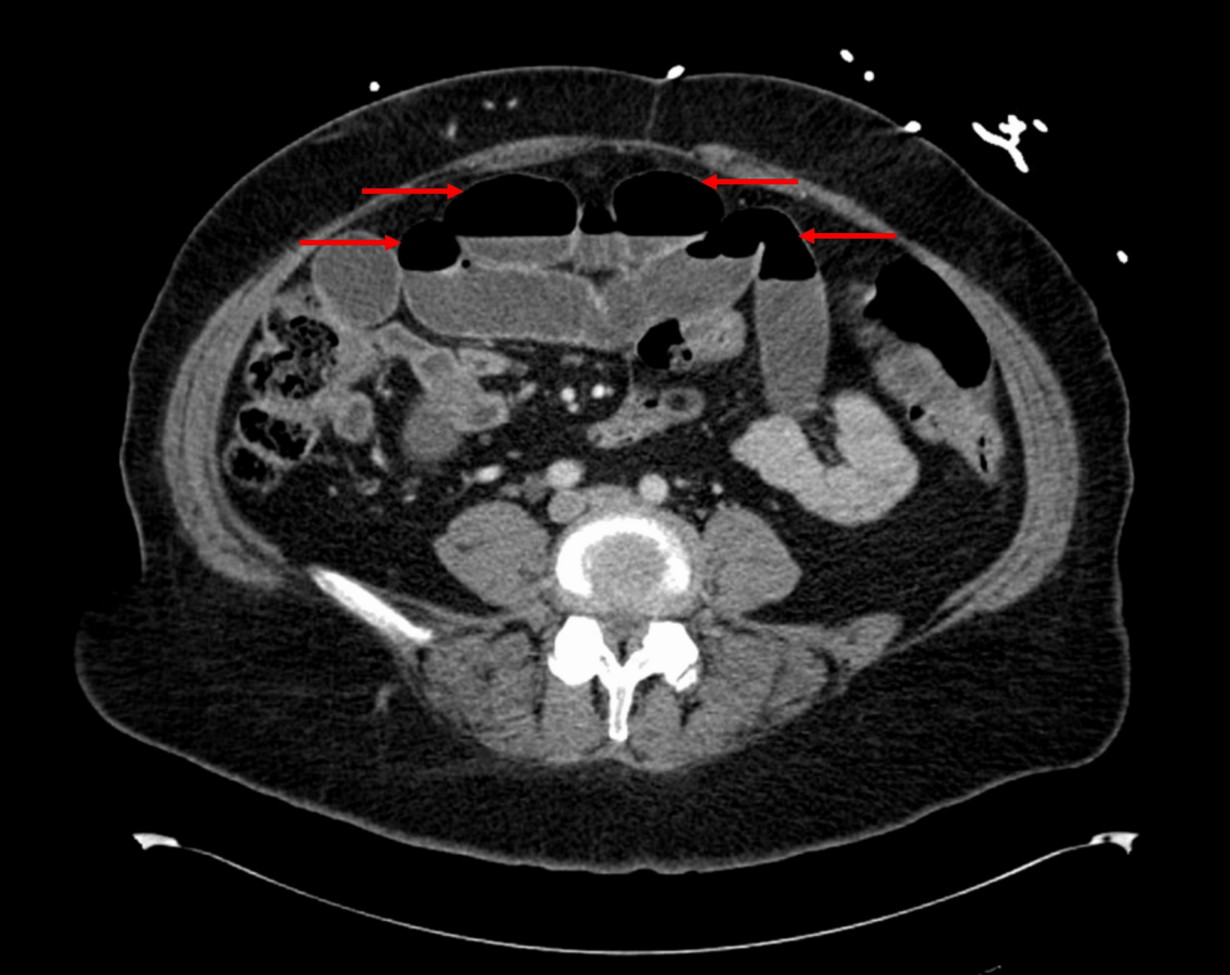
CT scan (axial view) showing dilated loops of bowels (shown by red arrows)
